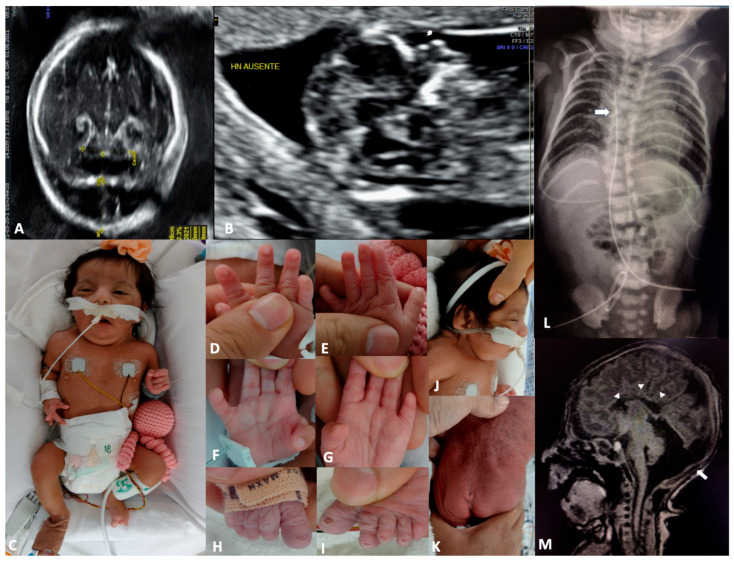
Clinical features in the *proposita*. (A,B) Prenatal ultrasound showing a cystic hygroma, mega cisterna magna, and absent nasal bone. (C) Coarse facial features. (D–I), Nail aplasia/hypoplasia, short fifth fingers. (J) Dysplastic, low-set ears, and redundant nuchal skin. (K) Deep sacral skin dimple. (L) Thorax radiography showing mild scoliosis and T6 hemivertebra (arrow). (M) Sagittal view of a brain MRI showing agenesis of the corpus callosum (arrowheads), severe hypoplasia of the cerebellum, and pseudocystic dilatation of the posterior fossa (arrow).
